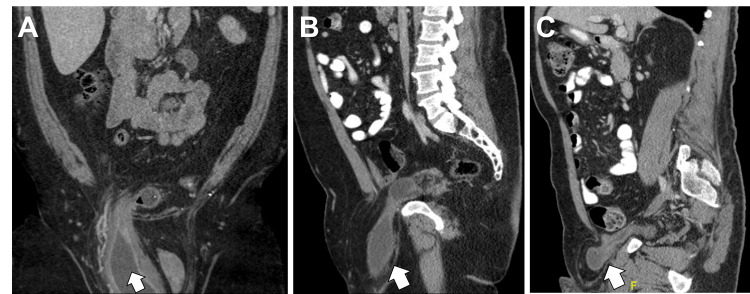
Preoperative CT images. Images of sliding inguinal bladder hernia (white arrows) of (A) a 72-year-old male (coronal view); (B) a 46-year-old male, and (C) a 68-year-old male (sagittal views).
